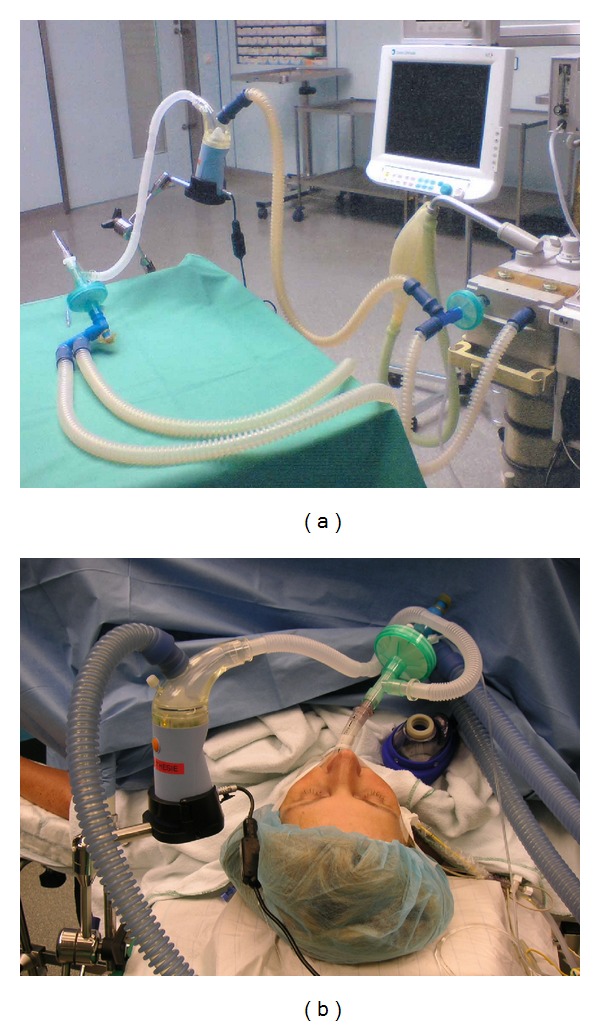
Assembly instruction for the integration of an ultrasonic nebulizer (Multisonic) in the ventilatory circuit. Intraoperative selective pulmonary vasodilation with inhaled iloprost.
